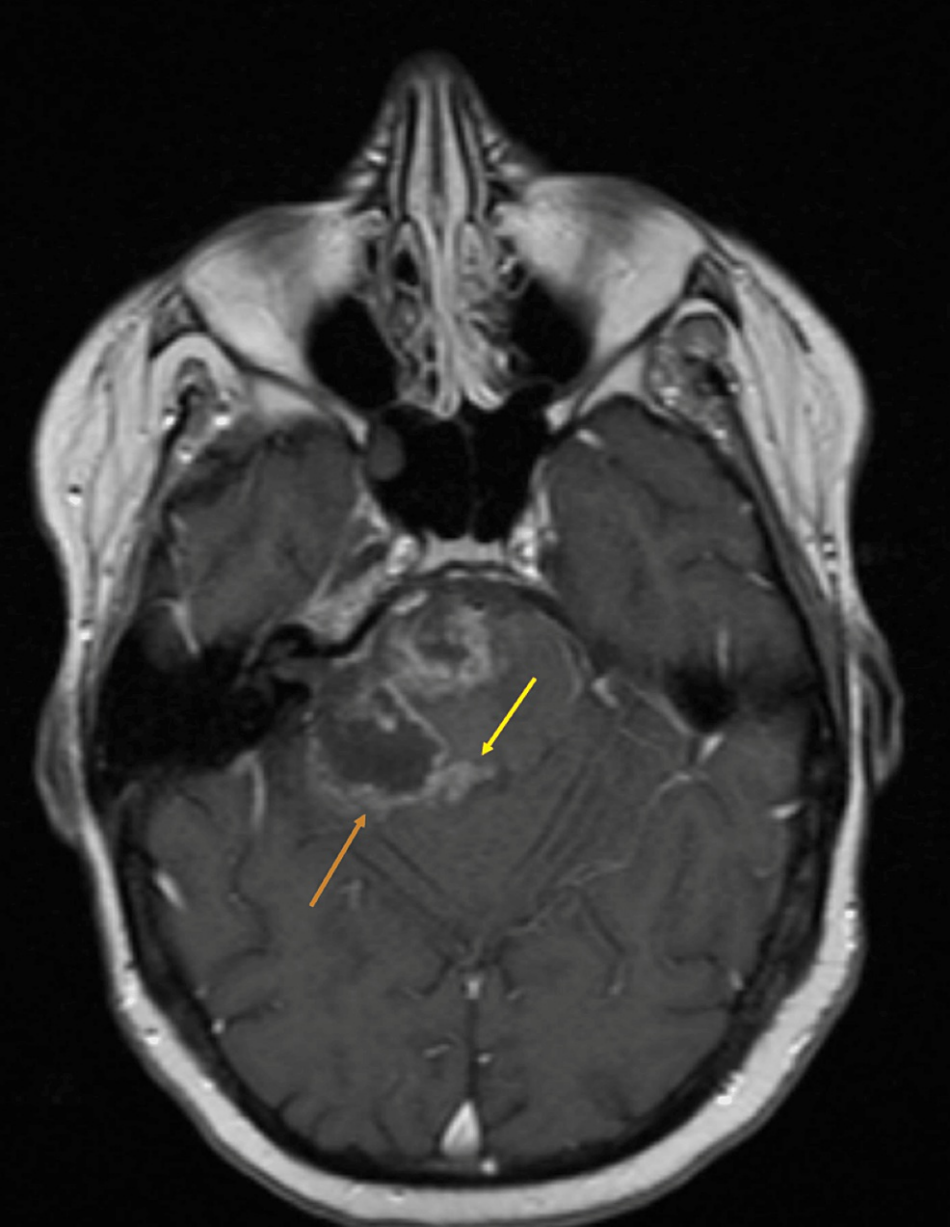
Axial T1 post-contrast MRI of the brain done to follow up previous non-contrast CT. The image shows an irregular area of rim enhancement with central low signal intensity secondary to high-grade tumor necrosis and known post-surgical changes (seen by the orange arrow). Additionally, there is also a midline region of nodular enhancement which may suggest tumor remnant/recurrence; this finding corresponds to the hyperdensity previously noted on CT (seen by the yellow arrow).
